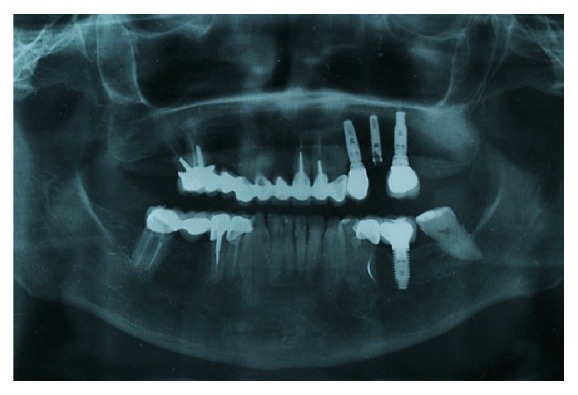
Panoramic radiograph. Implant (2.5) with fracture screw and hex devastated platform.
